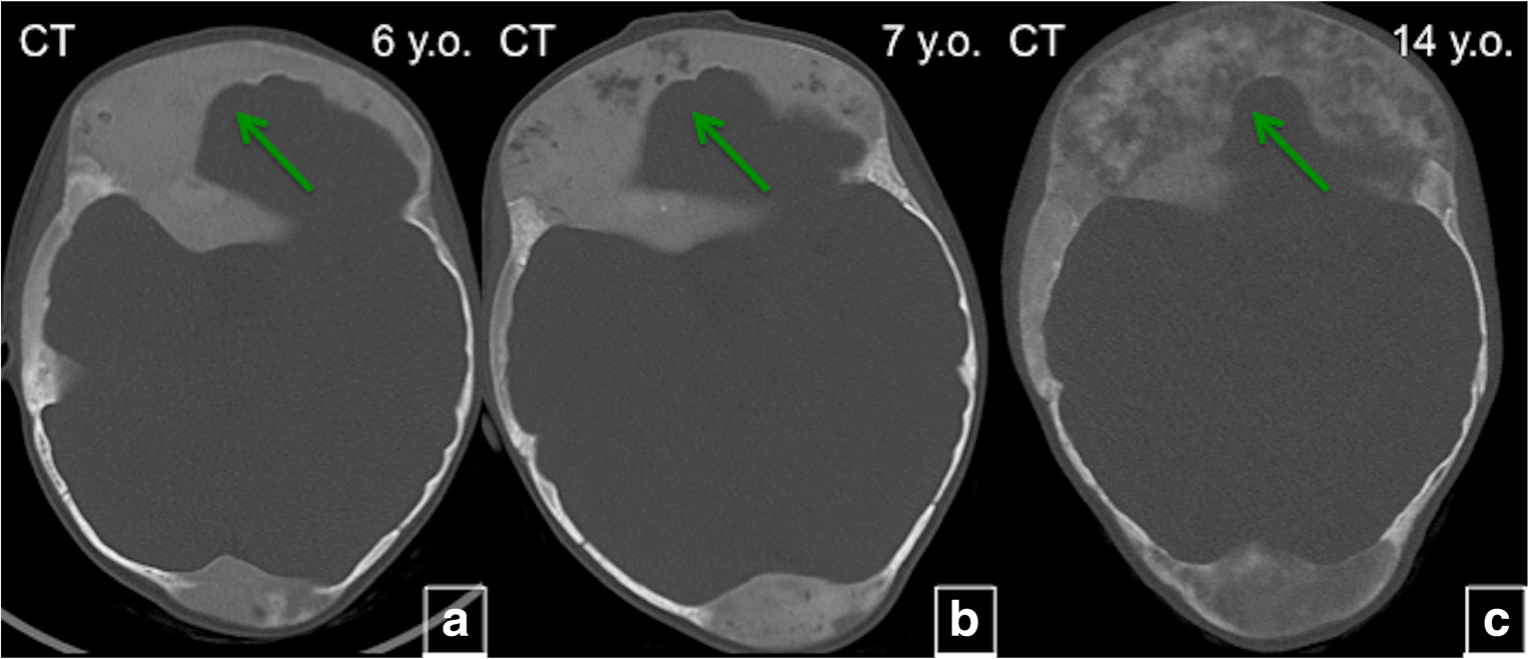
Age-related changes in fibrous dysplasia (FD). CT of the head on the same patient at age of 6 (a), 7 (b) and 14 years (c). Diffuse FD involvement with homogenous “ground glass” appearance (*green arrows*), which demonstrates the tendency to develop cystic lesions and become more heterogeneous with time (*green arrows*)
